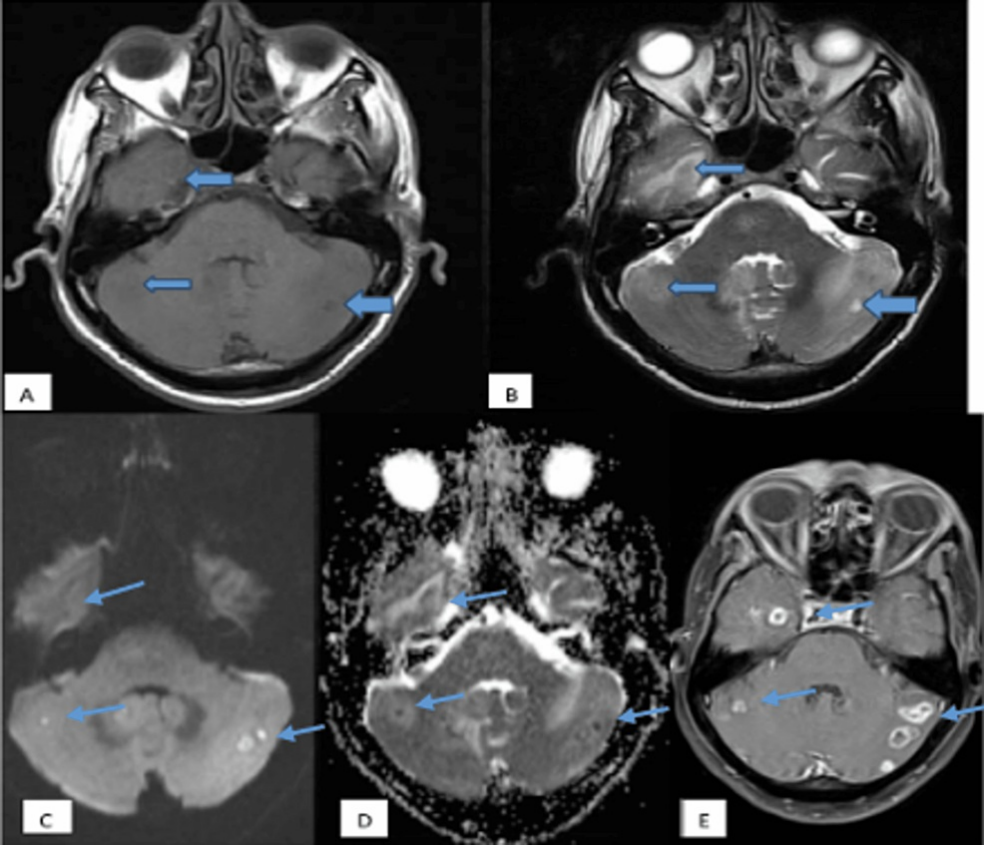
Tuberculoma - caseating granulomas with central liquefaction MRI images: (A) Axial T1WI, (B) axial T2WI, (C) axial DWI, (D) axial ADC map, and (E) axial T1-weighted post-contrast. These images show few, variable-sized, T1 iso and T2 hyperintense lesions with T2 hypointense rim (blue arrows) and moderate perilesional edema in bilateral cerebellar hemispheres and right temporal lobe with restricted diffusion on DWI. The ADC value obtained was 0.9 x 10^-3^ mm^2^/s. On post-contrast, the lesions show thick irregular ring enhancement. T1WI: T1-weighted imaging; T2WI: T2-weighted imaging; DWI: diffusion-weighted imaging; ADC: apparent diffusion coefficient.
